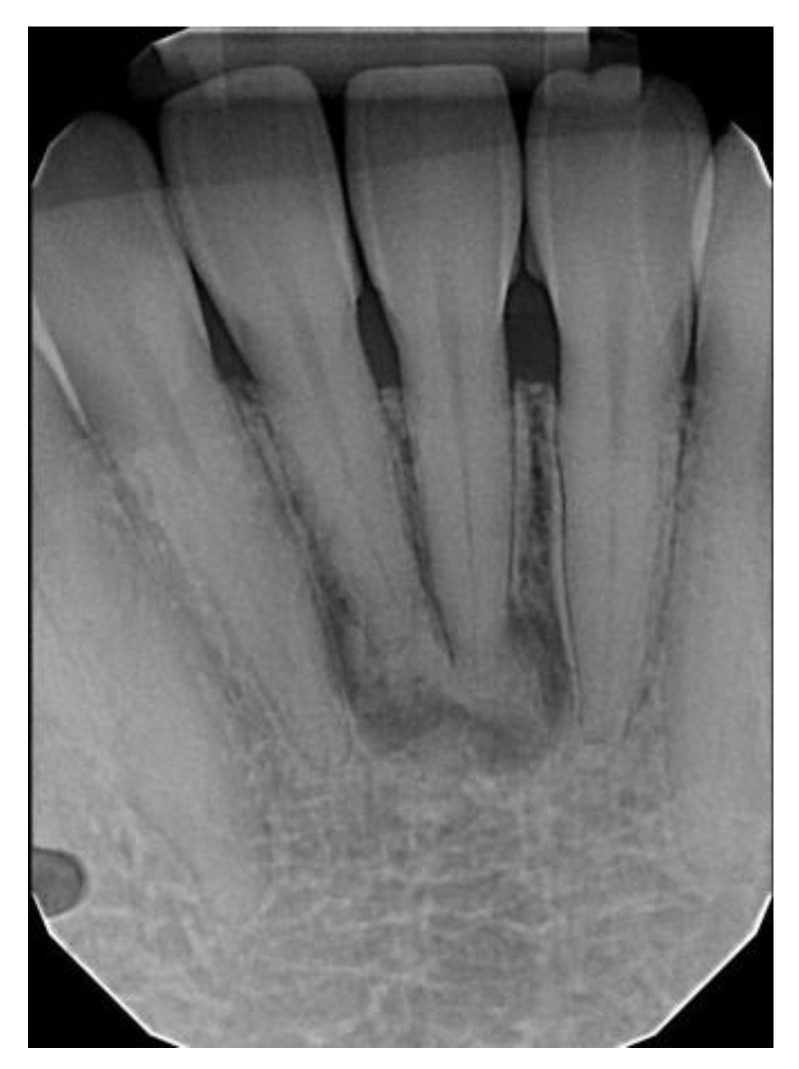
Periapical cemento-osseous dysplasia involving the central incisors of the mandible in an asymptomatic 45-year-old African American female.
